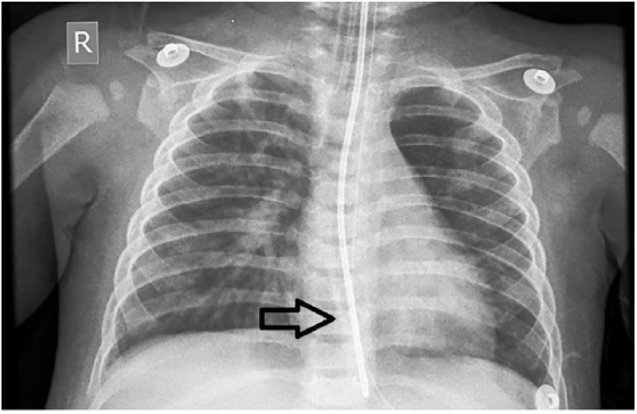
Chest X-ray showing increased chest wall edema leading to poor compliance with the esophageal catheter in situ (black arrow).
